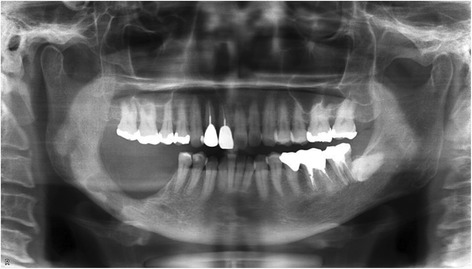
Preoperative panoramic radiograph in case 2. A radiolucent area was observed in the molar region of the right mandible because of recurrent squamous cell carcinoma
